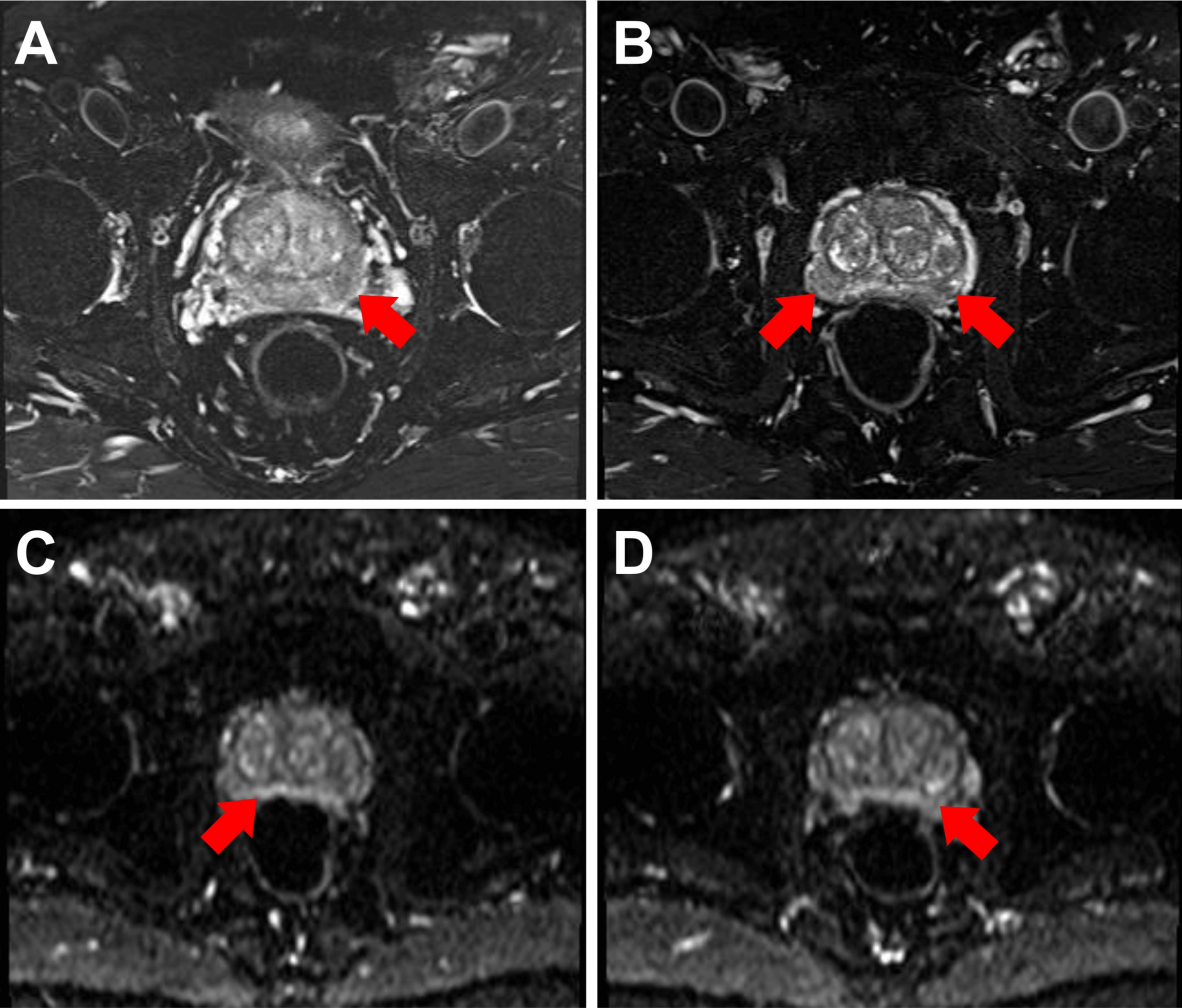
Preoperative multi-parametric MRI of the prostate (A-B) Axial T2-weighted images show hypointense lesions in the bilateral peripheral zones of the prostate (red arrows). (C-D) Diffusion-weighted images show enhanced signals in the bilateral peripheral zones (red arrows). MRI: magnetic resonance imaging
